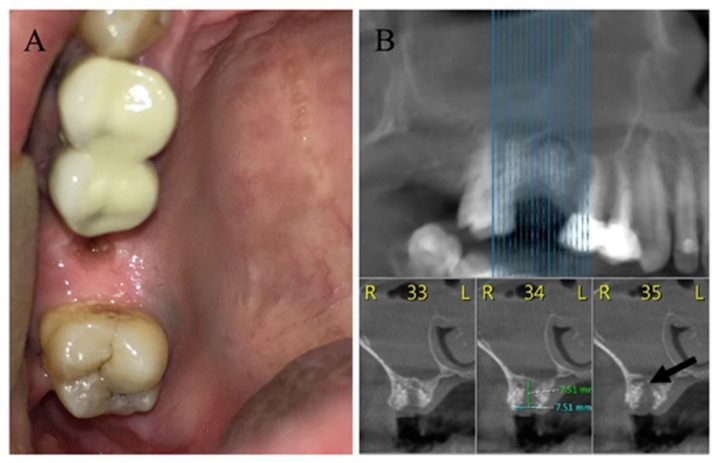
(A) Intraoral image of a 35-year-old smoker with an oroantral fistula (OAF) in the right posterior maxilla, secondary to removal of a failed dental implant and socket preservation. (B) Cone beam computed tomography (CBCT) of the patient presented in Figure 1A, demonstrating bony defect with discontinuity of the sinus floor (black arrow). The entire sinus is radio-opaque due to inflammation.
